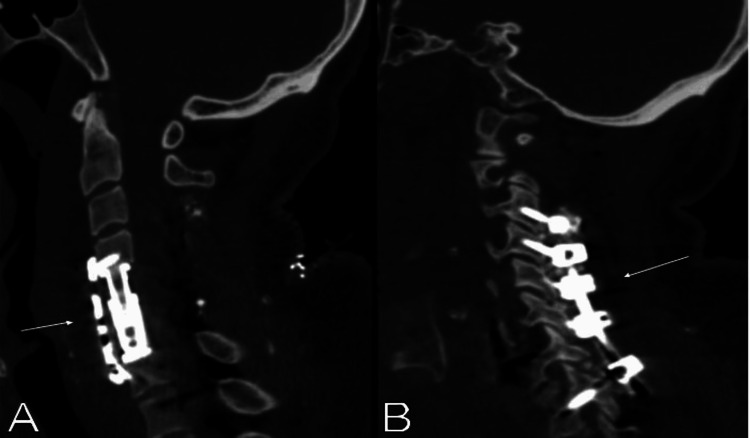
Cervical spine computed tomographic scan sagittal images showing the spinal reconstruction. (A) Anterior C5-C6 corpectomy with an expandable titanium vertebral body replacement cage from C4 to C7 and an anterior titanium plate (arrow) and (B) posterior C3-T1 instrumentation (arrow).
